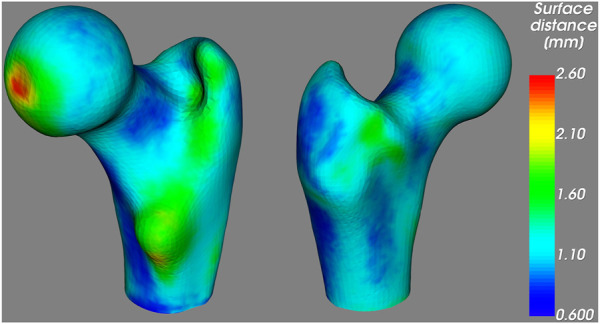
Average unsigned distance between the proximal femur surfaces obtained from the CT and the 3D DXA images, mapped on the standard femur.
